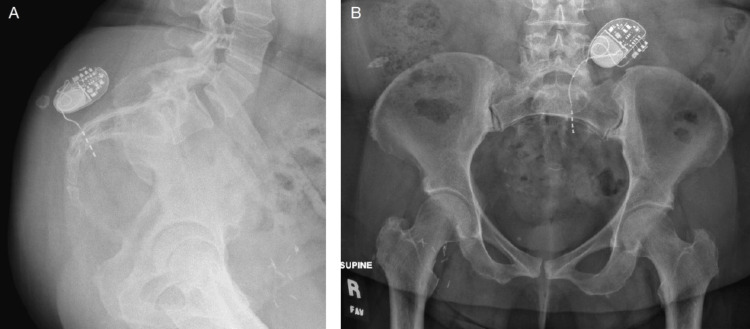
Pre-operative pelvic radiographs A) Lateral view demonstrates posterior lead migration. B) Anterior-posterior (AP) view demonstrates lead twisting near the generator.
